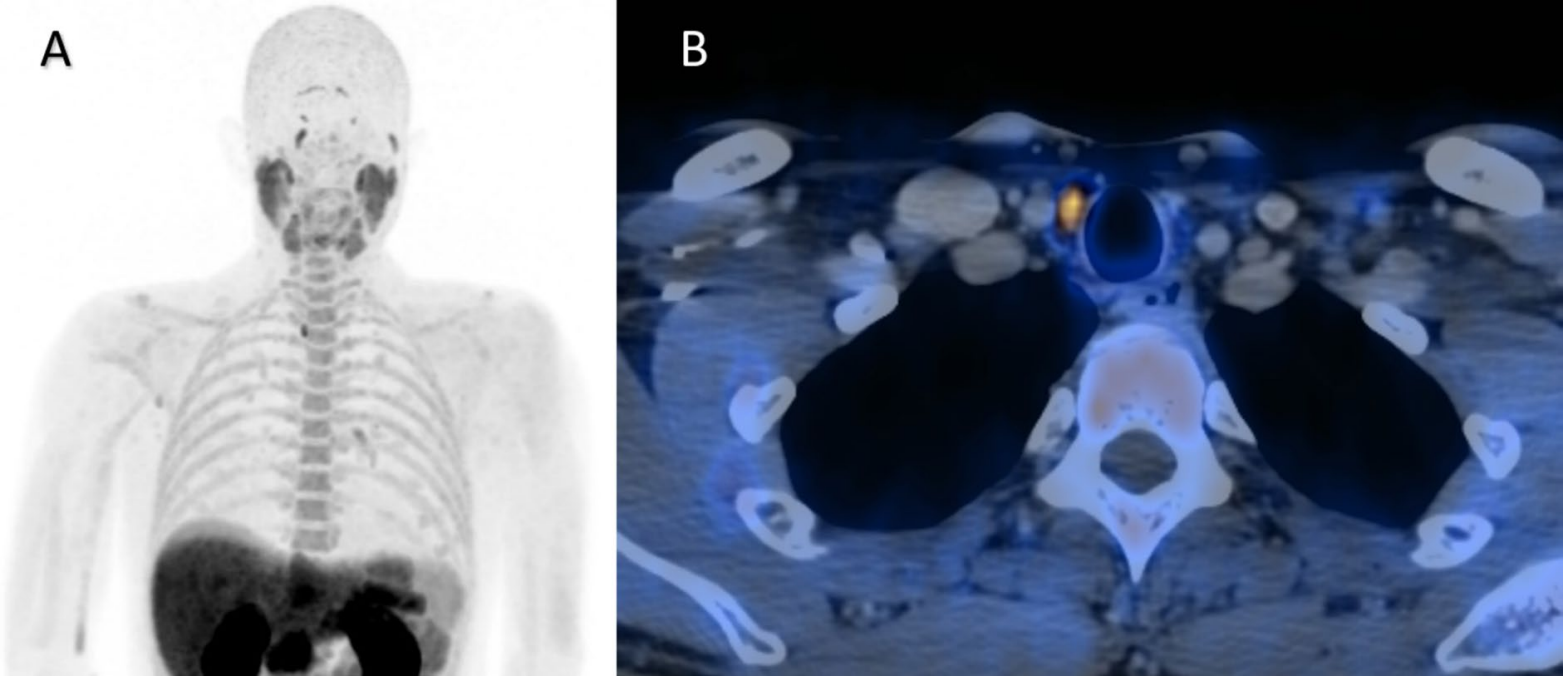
Right-sided orthotopic adenoma. Legend: 46-year-old man with pHPT, albumin-corrected serum calcium level 2.62 mmol/L (2.19–2.54 mmol/L), PTH 96.4 ng/L (15–65 ng/L). MIP (A) and contrast-enhanced axial PET/CT (B) image show a right-sided lower pole parathyroid adenoma (SUVmax 7.9, 11 × 7 × 5 mm), confirmed by surgery
